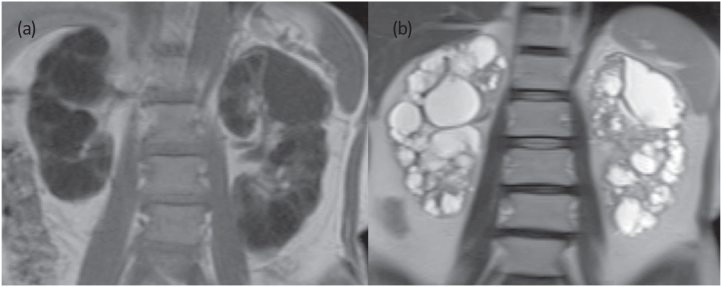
Magnetic resonance imaging of the kidney (coronal cross-sectional view). (A) T1-weighted image showing multiple cysts with uniform low intensity (arrow) in both kidneys and (B) T2-weighted image showing multiple cysts with uniform high intensity (arrow) in both kidneys.
